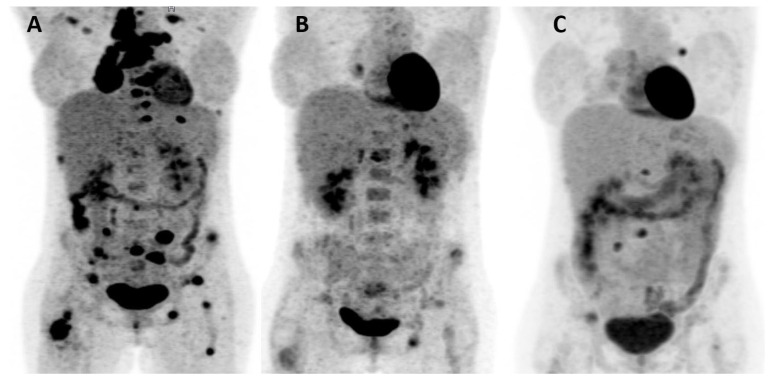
PET-CT prior to treatment (A), showing remarkable response after 2 cycles of chemoimmunotherapy and multi-site radiotherapy (B) and slight progression with a new PET-avid left hilar lymph node and a bone metastasis in L3 (C).
